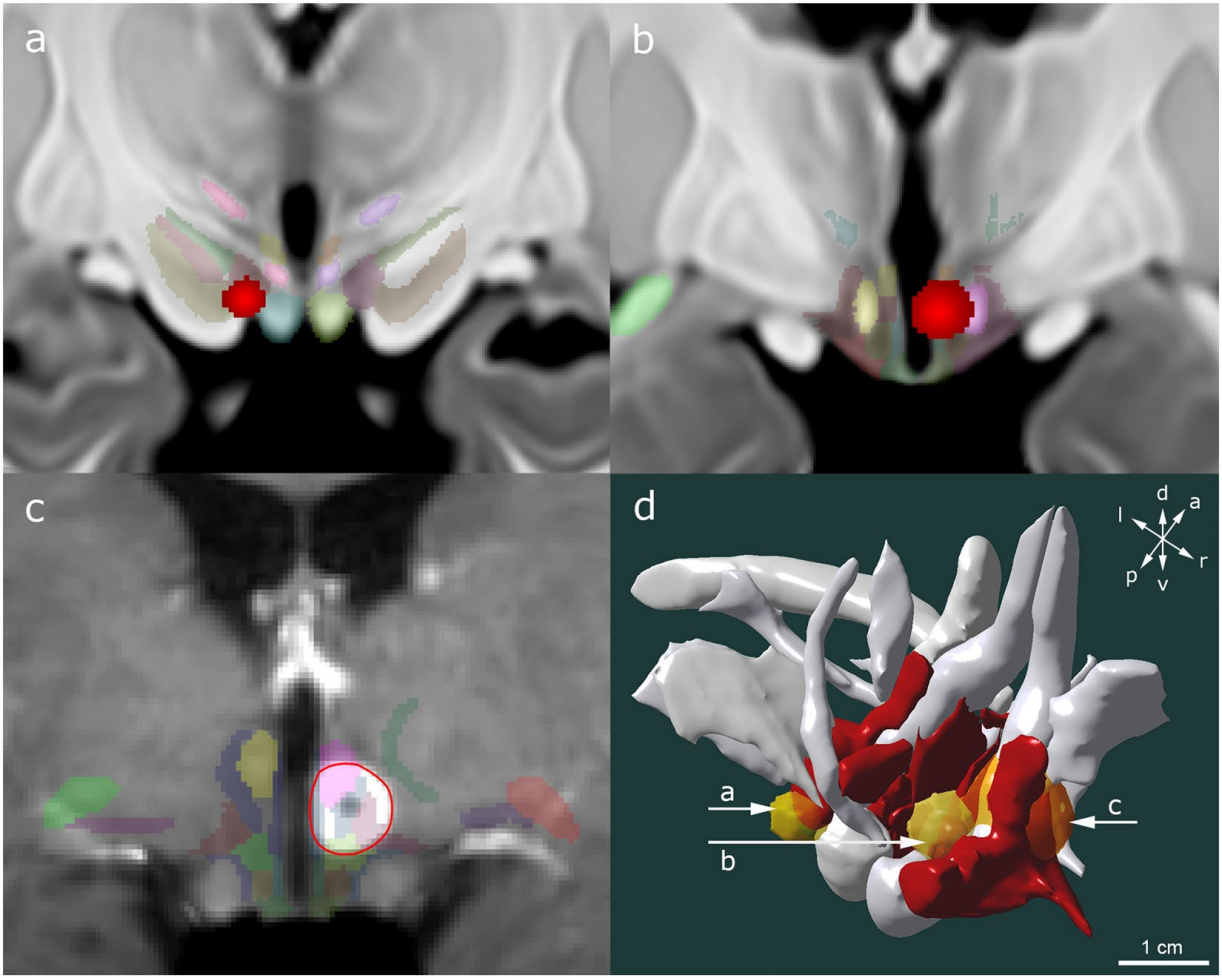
Clinical application of the hypothalamic atlas. Volumes of tissue activated (VTAs, shaded red) of patients receiving (a) hypothalamic deep brain stimulation (DBS) in the treatment of morbid obesity and (b) forniceal stimulation in Alzheimer’s disease. VTAs were overlaid on representative coronal sections from the T1w high-resolution MRI template. The superimposed hypothalamic atlas depicts the neural substrate encompassed during electrical stimulation. (c) An incidental, contrast-enhancing solitary hypothalamic metastasis (red circle) in a patient who had previously been diagnosed with a melanoma. The patient’s T1w MRI scan was registered with the high-resolution template and the atlas superimposed. (d) Oblique view of a hypothalamic 3D reconstruction featuring the spatial relationship between VTAs (shades of yellow), metastasis (shades of orange), hypothalamic nuclei (red), and surrounding structures (gray). The posterior hypothalamus (PH) was removed on the right side to allow visualization of VTAs.
